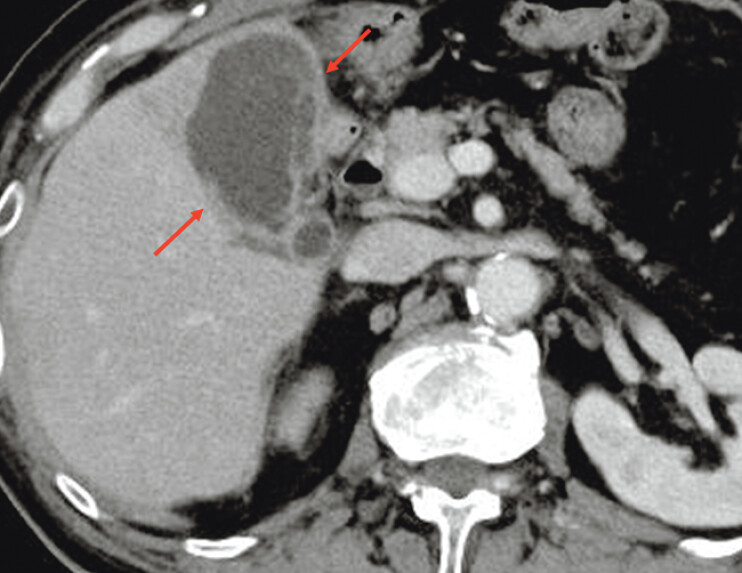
Computed tomography revealing an enlarged gallbladder with wall thickening (red arrow), indicating acute cholecystitis.
